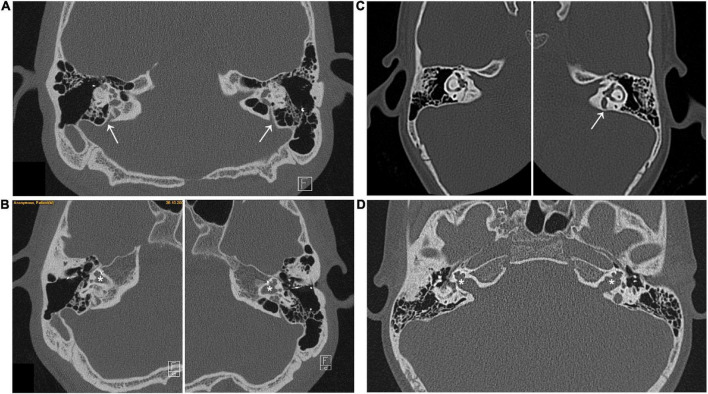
Axial CT scan of the index patients showing both sides. (A,B) Patient #569, arrows in panel (A) indicate the bilateral enlargement of the vestibular aqueduct (EVA), * in panel (B) indicate the bilateral incomplete partition of the cochleae with missing modiolus (IP3), a typical hallmark of POU3F4-related deafness. (C,D) Patient #667, arrow in panel (C) indicates the EVA on the left side, while on the right side the vestibular aqueduct appears normal; * in panel (D) indicate the bilateral IP3.
